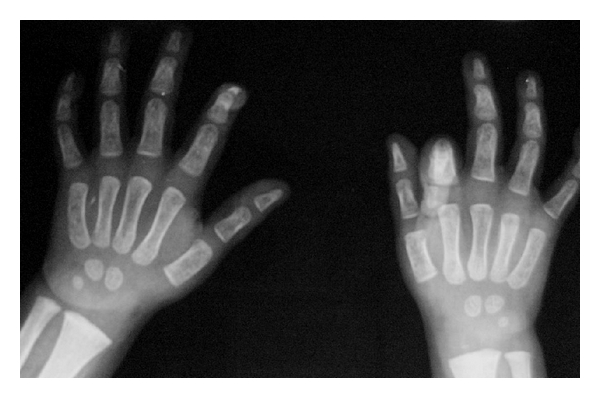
Plain radiographs showing little cortical hyperdensity of right hand fourthmetacarpal.
